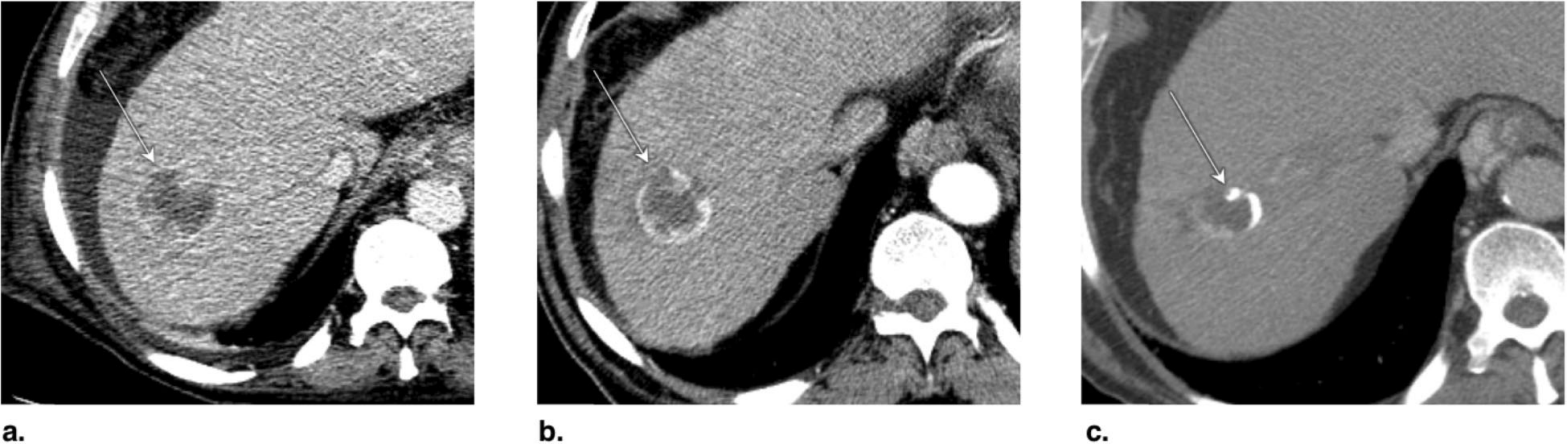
Axial CT obtained (a) pretreatment: image in portal venous phase shows enhancing “capsule” and washout of a LR-5 observation in segment VII. b 4 months post drug-eluting beads TACE (DEB-TACE): image in late arterial phase shows thicker peripheral arterial phase hyperenhancement (arrow). The treated observation is categorized LR-TR viable. The lesion was subsequently treated with transarterial chemoembolization (TACE). c Axial-unenhanced CT post TACE: image shows the expected ethiodized oil retention (arrow)
